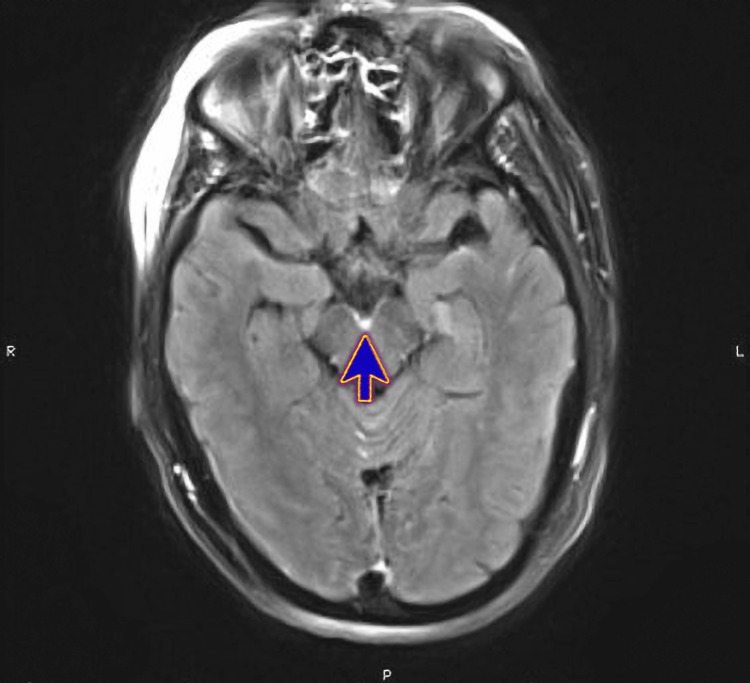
Magnetic Resonance Imaging (MRI) of the brain showing leptomeningeal enhancement along with interpedicular involvement with suspected cervical ependymal enhancement (blue arrow)
